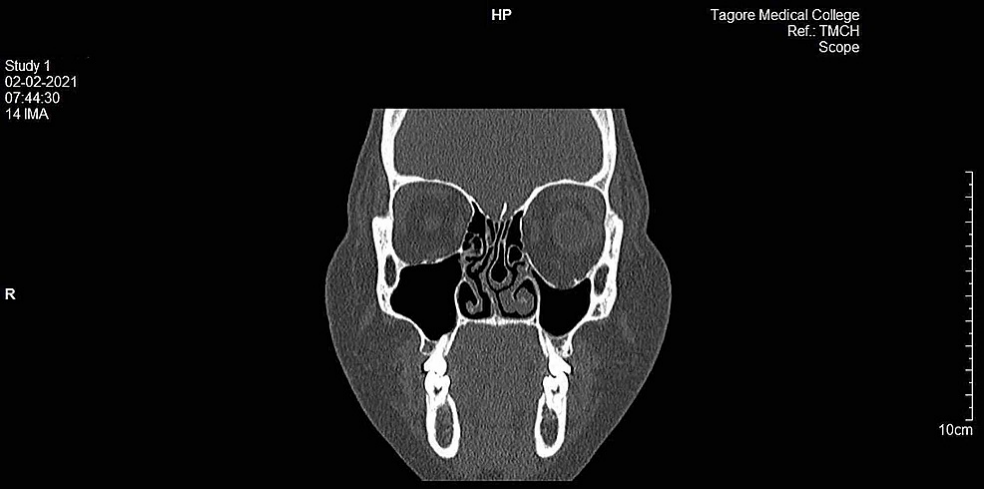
Postop 22-month CT scan (coronal): The orbital contents repositioned within the orbit and the floor has been reconstructed with cartilage graft
